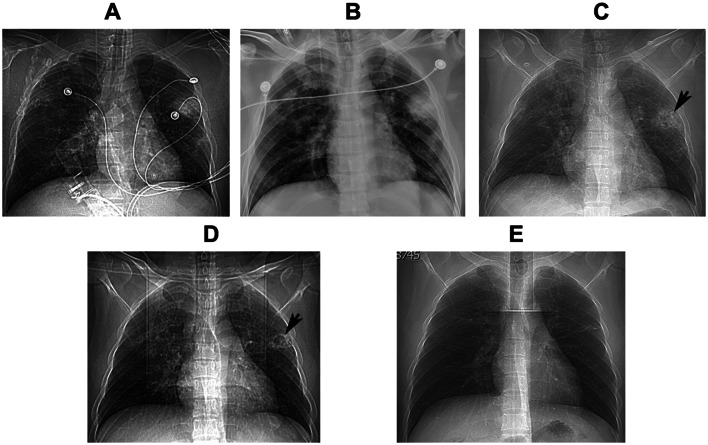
Plain chest radiographs. (A) On the 1st day after admission. (B) On the 4th day after admission. (C) Multiple small cavitations in nodular opacities in right upper lung (black arrow) observed on the 8th day after admission. (D) A large cavitation formed in right upper lung (black arrow) observed on the 15th day after admission (discharge). (E) one month later after discharge
